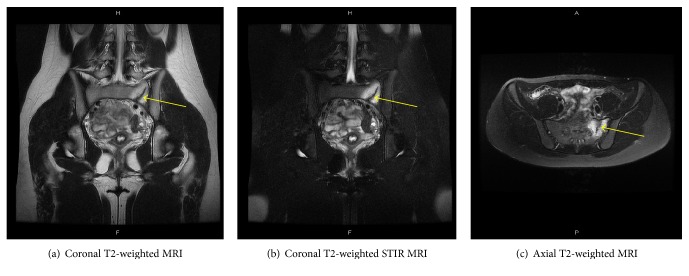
MRI T2 and T2 short tau inversion recovery (STIR) scan shows a linear area of decreased signal intensity of left sacrum with surrounding a T2 hyperintense area (arrow).
